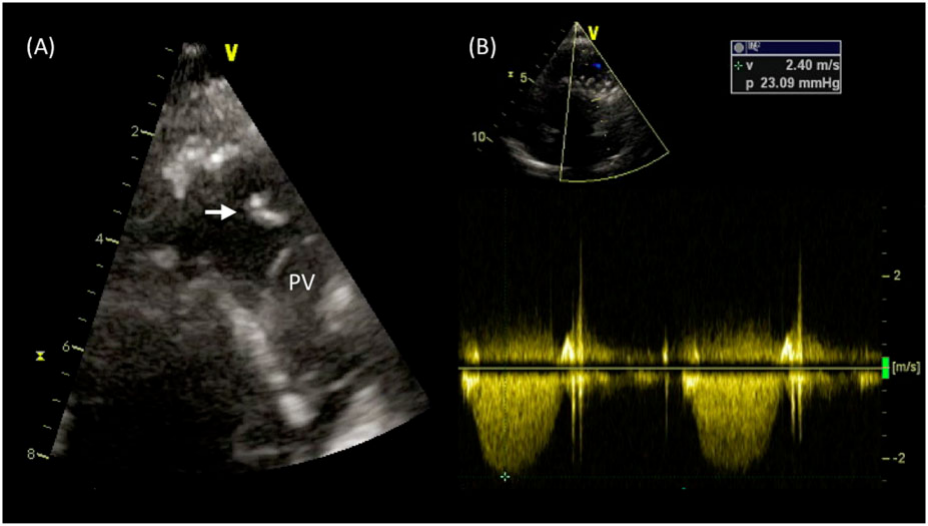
A case of pulmonary valve endocarditis in a patient with double outlet right ventricle and pulmonary stenosis after percutaneous pulmonary valve implant (Case 1). (*A*) High parasternal echo view: a mobile filamentous echogenic mass attached to the struts of the proximal segment of the stent in right ventricular outflow tract is visualized. (*B*) Continuous-wave Doppler signal demonstrating normally functioning pulmonary valve. Arrow, vegetation; PV, pulmonary valve; RVOT, right ventricular outflow tract.
